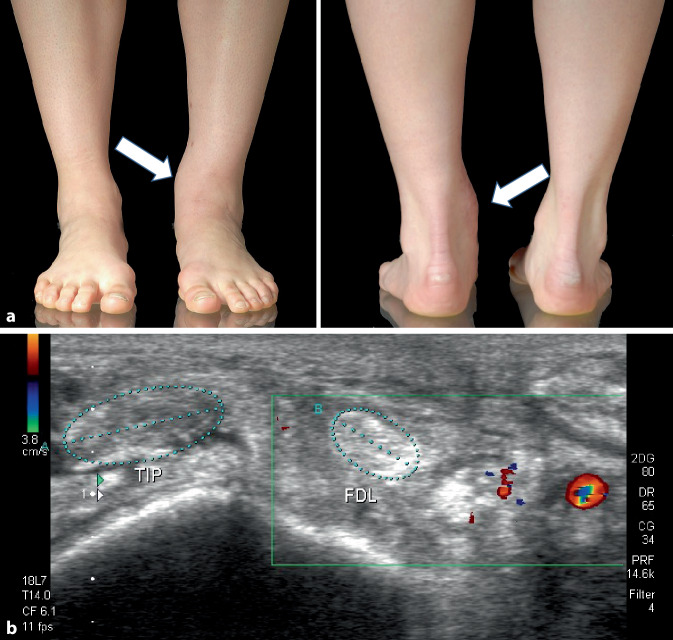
a Klinisch verstrichene Kontur des Innenknöchels (*Pfeile*) bei luxierter M.-tibialis-posterior-Sehne b Koronarer Sonographieschnitt auf Höhe der nach ventral breit ausgezogenen M.-tibialis-posterior-Sehne (*TIP*), in situ befindliche M.-flexor-digitorum-Sehne (*FDL*)
